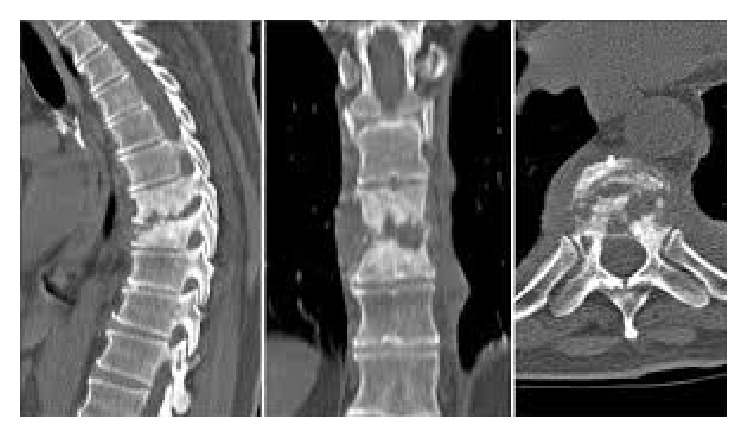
CT thoracic spine shows T10 vertebral body destruction.
